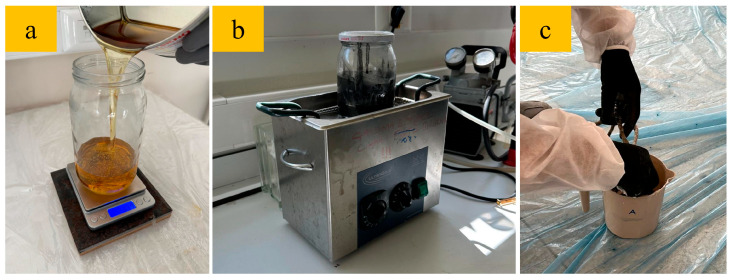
Preparation of GNP–epoxy resin system: (a) weighing and (b) ultrasonic mixing of GNP/hardener mixture and (c) the final mixture of GNP–hardener–epoxy resin prepared for the wetting of fiber ropes.
